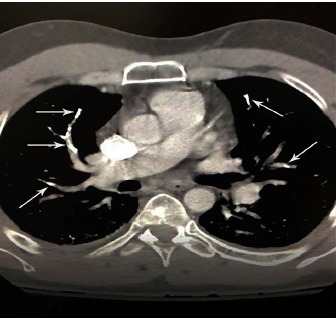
Computed tomographic angiogram of the chest of a 43-year-old male depicting hyperdense material in distal pulmonary arteries (arrows) consistent with cement emboli.
